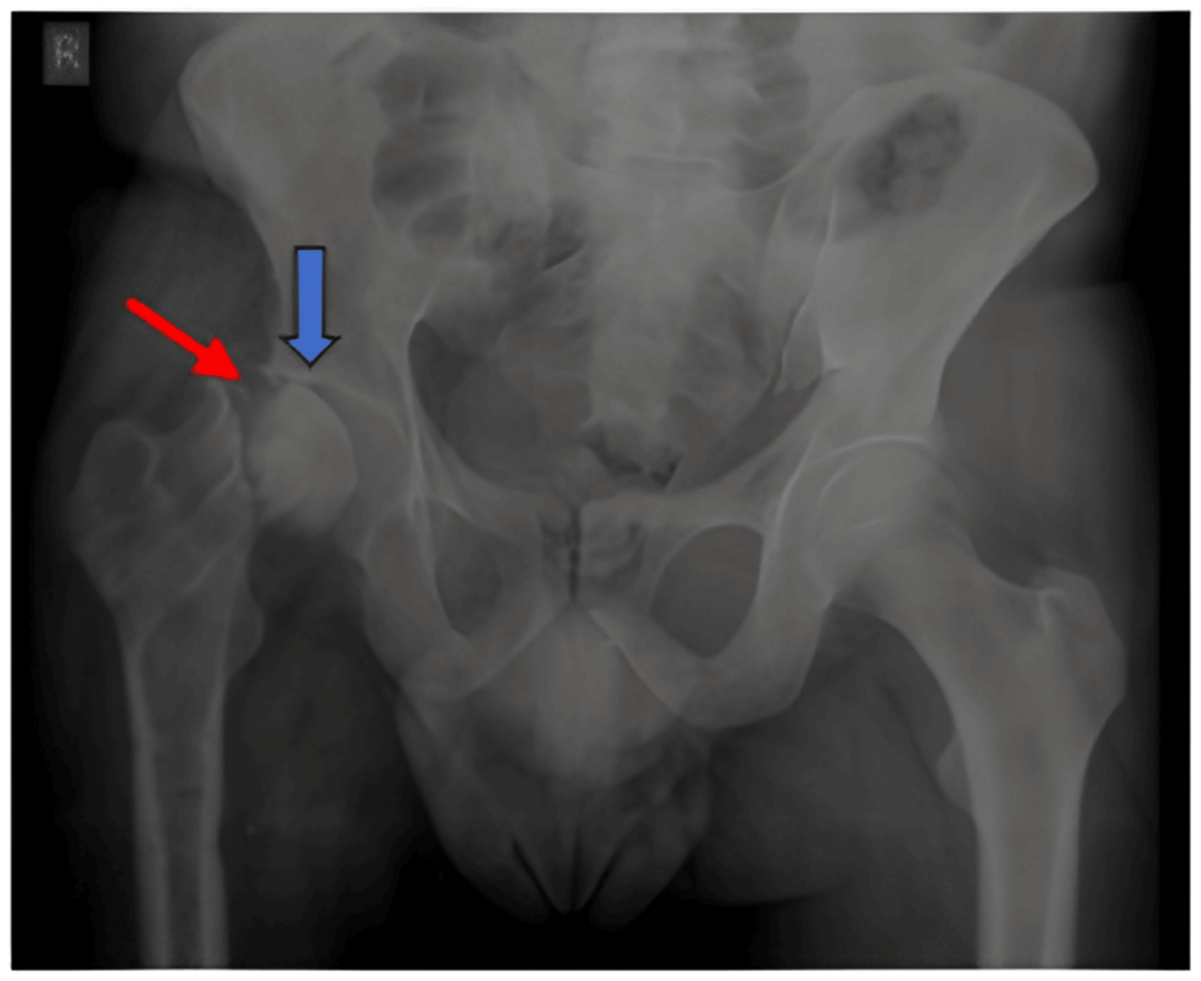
X-ray of the pelvis and both hips of case 2, AP view. Findings:* *A fracture of the neck of the right femur is noted (red arrow) to rule out the nonunion of the fractured neck. Opposed fractured margins show irregular areas of sclerosis. Marginal osteophytes are noted at the fractured right head and acetabulum. The right femoral head shows increased density or sclerosis with a loss of normal contour and secondary degenerative changes, representing Ficat and Arlet stage IV AVN (blue arrow). Increased joint space is noted at the right hip joint. Internal fixation markings are noted at the intertrochanteric region and proximal femur on the right side. AP, anteroposterior; AVN, avascular necrosis
